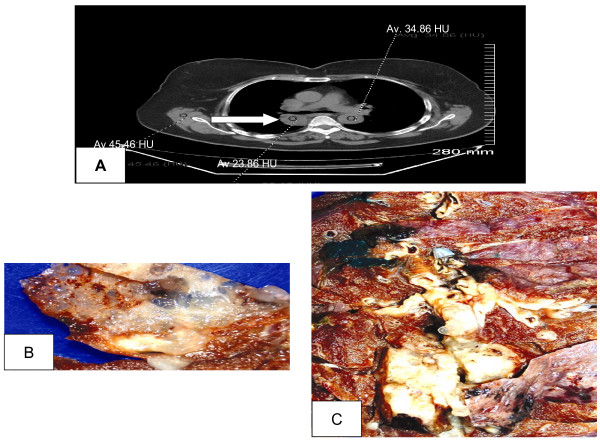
(A) Computed tomography scan shows a well-defined mass (white arrow) with slightly lobulated margins posterior to the right hilum. The annotations show comparative attenuations within the mass (23.86 HU), aorta (35 HU) and skeletal muscle (46 HU), indicating a cystic lesion. (B) Gross photograph demonstrating a nodular cystic mass. (C) Gross photograph demonstrating translucent cystic appearance due to the abundant mucin content.
